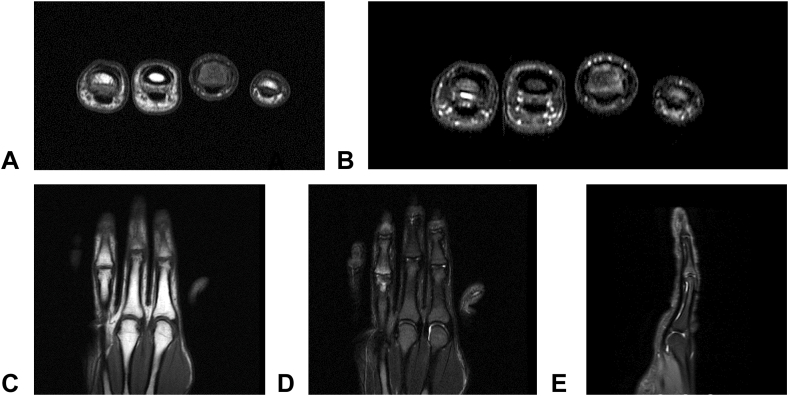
MRI demonstrating intact collateral ligaments and continuity of both the dorsal (central slip) and volar (volar plate) fragments.
